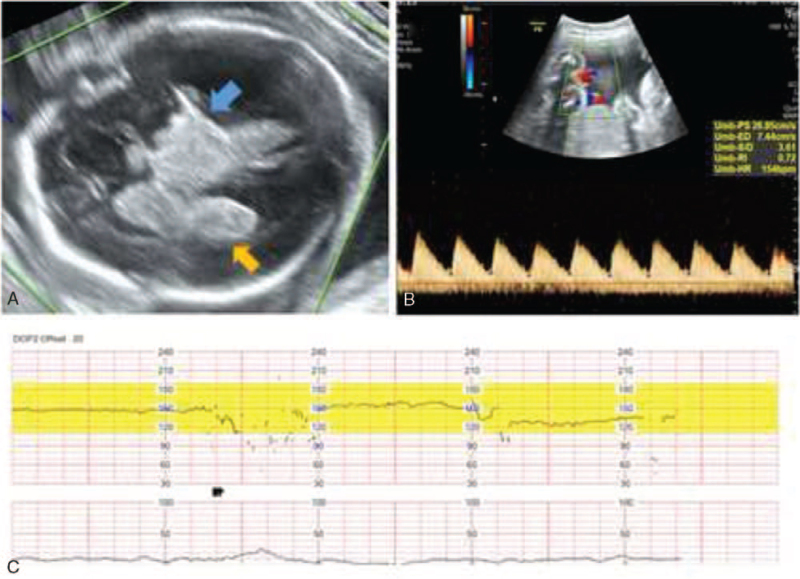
At the diagnosis of fetal brain hemorrhage. (A) Ultrasonography of fetal brain showing high echogenicity in the intracranial space, suspicious of fetal intracranial hemorrhage. Yellow arrow indicates intraventricular hemorrhage, blue arrow indicates thalamic hemorrhage. (B) Umbilical artery velocity wave S/D ratio was 3.2 to 4.0. (C) Nonstress test (NST) showed deceleration of fetal heart rate.
